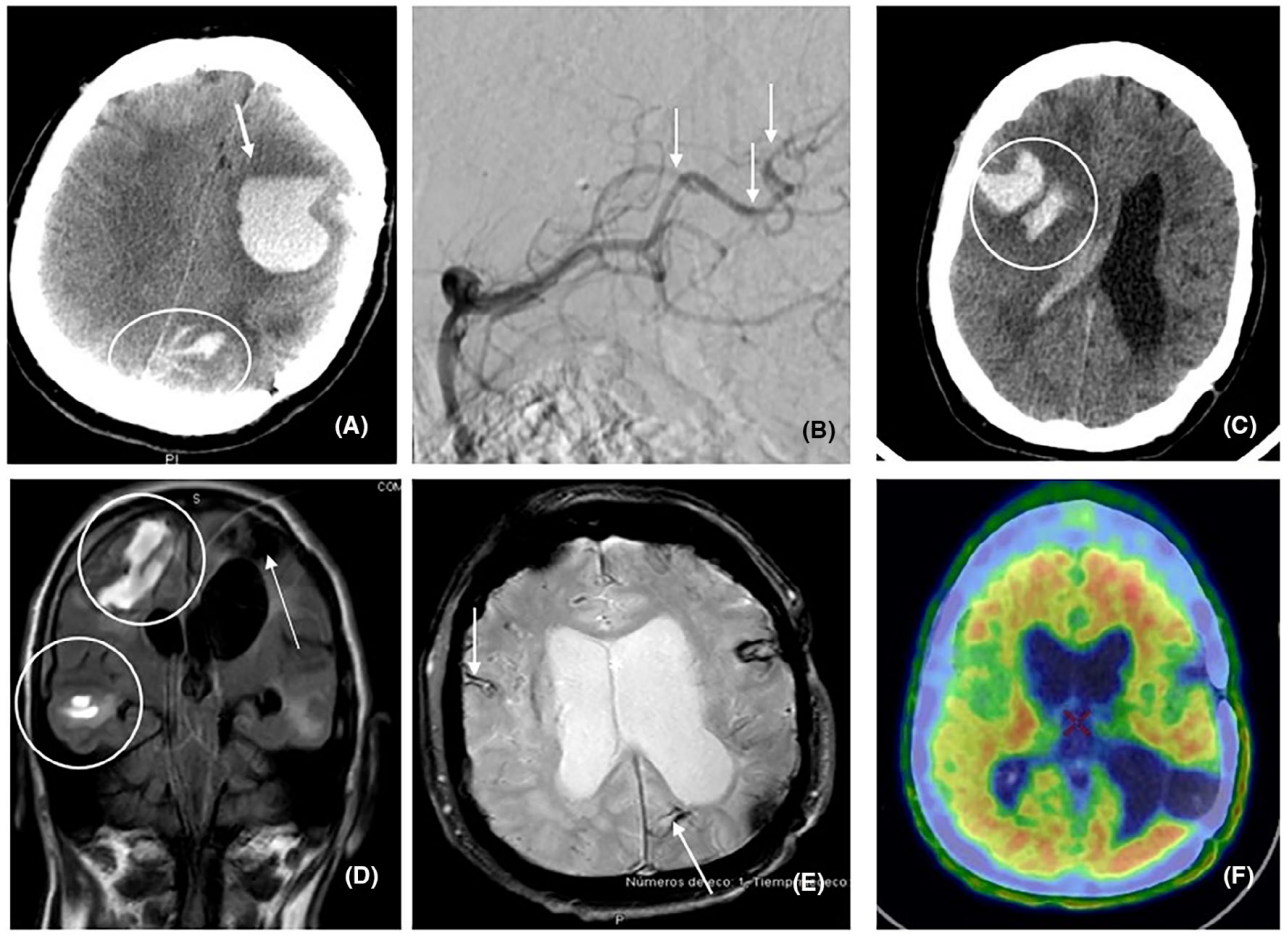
(A) Axial CT scan showing a left frontal hematoma (arrow), with surrounding edema. There is also a focus of SAH in the ipsilateral parietal convexity sulci (circle). (B) Cerebral angiography with selective run of the left vertebral artery, showing multiple changes and irregularities in the parito‐occipital branches of the posterior cerebral arteries (arrows). (C and D) Axial section of noncontrast CT (C) and coronal FLAIR MRI (D) showing an acute, heterogeneous, right frontotemporal hematoma (circles). The FLAIR image also shows sequelae of the previous hemorrhagic lesion (arrow), together with diffuse alteration of the white matter signal. (E) MRI, FFE T2* sequence showing sequelae of lobar intraparenchymal hemorrhages, together with cortical siderosis (arrows) and small microbleeds. (F) Axial section of 18F‐florbetaben PET‐CT scan. Isotope uptake in all the cortical reference areas.
